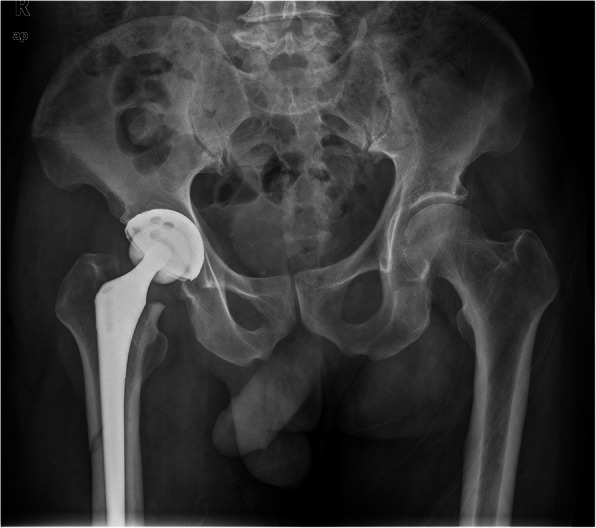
Anteroposterior radiograph of the right hip in postoperative day 1 showed the distal extension of the fracture line down the lateral cortex
